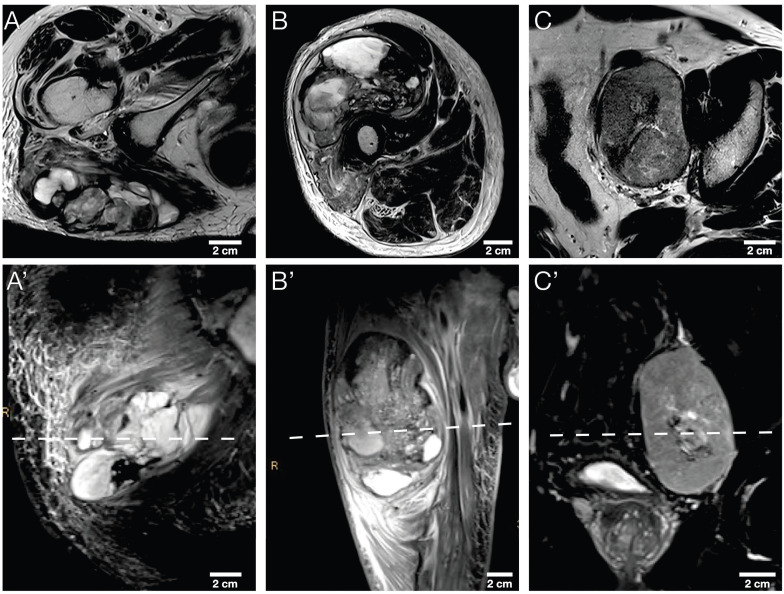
Morphological MRI sequences of the three STS patients. Exemplary axial T2w images (A, B, C) and corresponding coronal T2w-STIR images (A', B', C') of the three STS patients included. The dashed line in the lower row indicates the sectioning plane of axial T2w images in the upper row. STIR, short tau inversion recovery.
